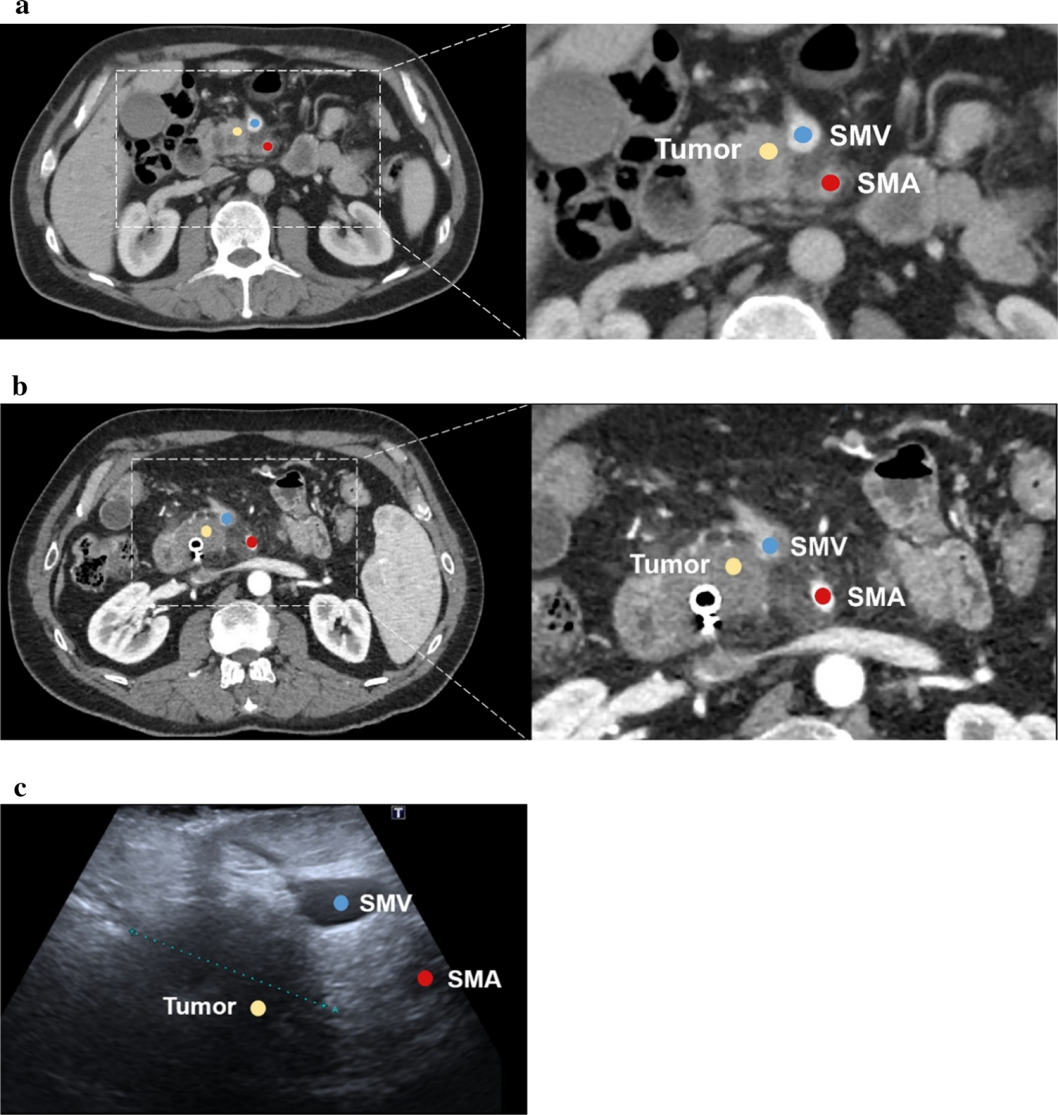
Typical example of downstaging after IOUS. a CT imaging at time of diagnosis (before biliary stent placement): involvement of the superior mesenteric artery (SMA; 270–360°) and superior mesenteric vein (SMV; 90–180°). Resectability status at diagnosis: locally advanced (LAPC). b CT imaging after completing preoperative chemoradiotherapy: persistent infiltration was seen surrounding the SMA (> 270°) and the superior mesenteric vein (90–180°), essentially unchanged when compared with initial imaging. Staging after preoperative therapy: LAPC. c Intraoperative ultrasound (IOUS): at most, 90° contact with the superior mesenteric vein was visible and no involvement of the superior mesenteric artery. Staging after IOUS: resectable. Time between last preoperative imaging and IOUS: 35 days
